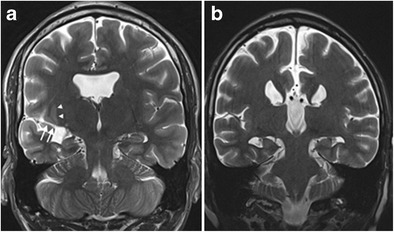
IHI associated with other developmental anomalies. Coronal T2-weighted image in a 22-year-old male patient with mental retardation and epilepsy (a) shows incomplete inversion of the right hippocampus (white asterisk at the collateral sulcus) along with a right perisylvian open lip schizencephaly (*white arrows*), right perisylvian subependymal heterotopia (*white arrowheads*) and agenesis of the septum pellucidum. Coronal T2-weighted images in a 25-year-old patient with mental retardation and epilepsy (b) shows agenesis of the corpus callosum and an abnormal globular shape of both hippocampi, corresponding to a bilateral incomplete hippocampal inversion
